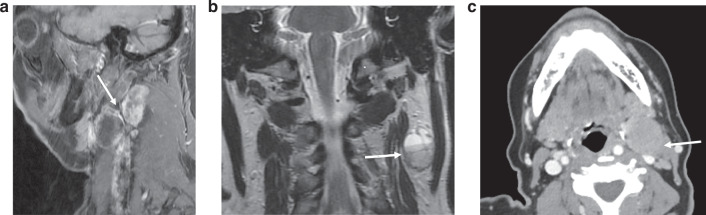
Principles to promote inter-rater agreement in iENE assessment. a Grade 1 iENE: Sagittal T1FS+c MRI– showing two adjacent metastatic Level II + III nodes with irregular nodal margins and perinodal fat stranding, but with a clear fat plane separating the 2 nodes. These nodes had appeared to be coalescent on axial images but review of coronal + sagittal imaging planes resulted in Grade 1 iENE. b Grade 0 iENE: Coronal T2 MRI showing cortical lobulation within a cystic metastatic Level II node (arrow), lobulation of a single metastatic node should be should be distinguished from coalescence of adjacent nodes to avoid overcalling Grade 2 iENE. This node has a well-defined margin and there is no perinodal fat stranding, therefore Grade 0 iENE. c Grade 0 iENE: Axial CECT demonstrating loss of the fat plane between a metastatic Level II node and sternocleidomastoid muscle (arrow). However, there is no extranodal enhancement to indicate muscle invasion of the muscle (compare with Fig. 2d), therefore Grade 0 iENE.
